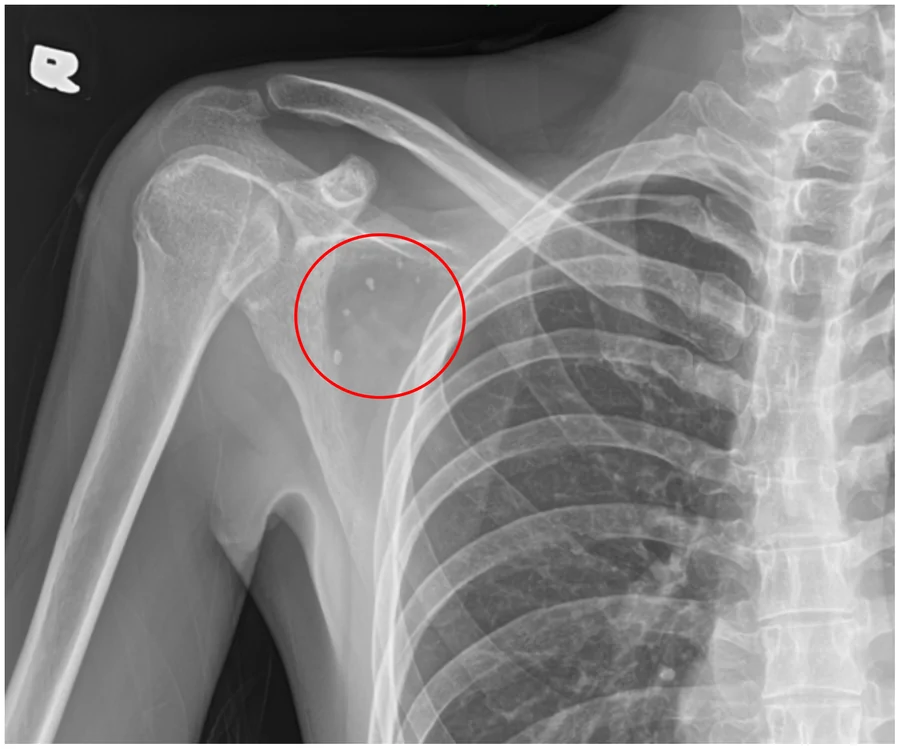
Plain radiograph of the right shoulder demonstrated multiple calcific densities consistent with vascular phleboliths (red circle) suggestive of venous malformations.
